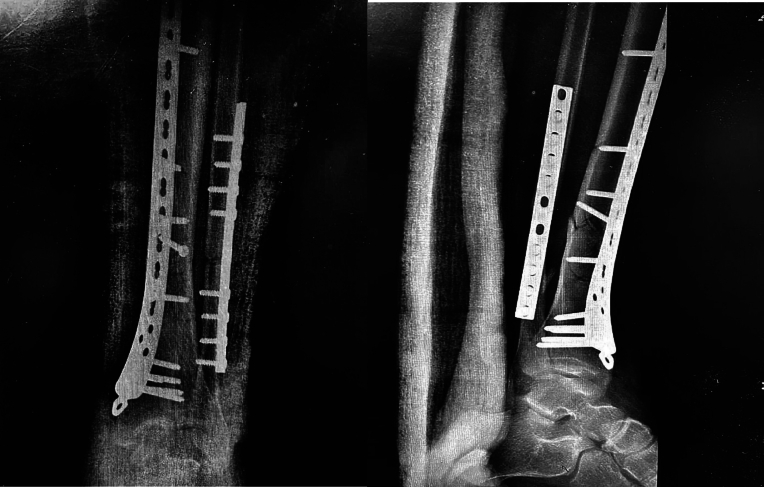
Postoperative anteroposterior and lateral radiographic imaging showing fracture at the junction of the middle and distal third of his tibia.
